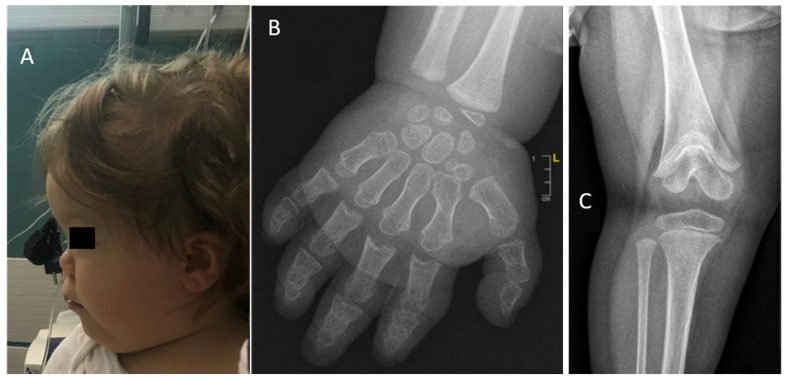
(A) Profile photograph of individual V.I at 2 years old showing features similar to photograph (A) as well as low-set ears. (B) Radiograph of the right hand of individual V.1 at 18 months showing acrodysostosis, brachymetacarpy and brachydactyly, cone-shaped epiphyses. Bone age is also very advanced (about 5 years in this radiograph). (C) Radiograph of the right knee of individual V.1 at 18 months showing cup-shaped metaphyses with embedded epiphyses (specific radiological appearance of acroscyphodysplasia).
